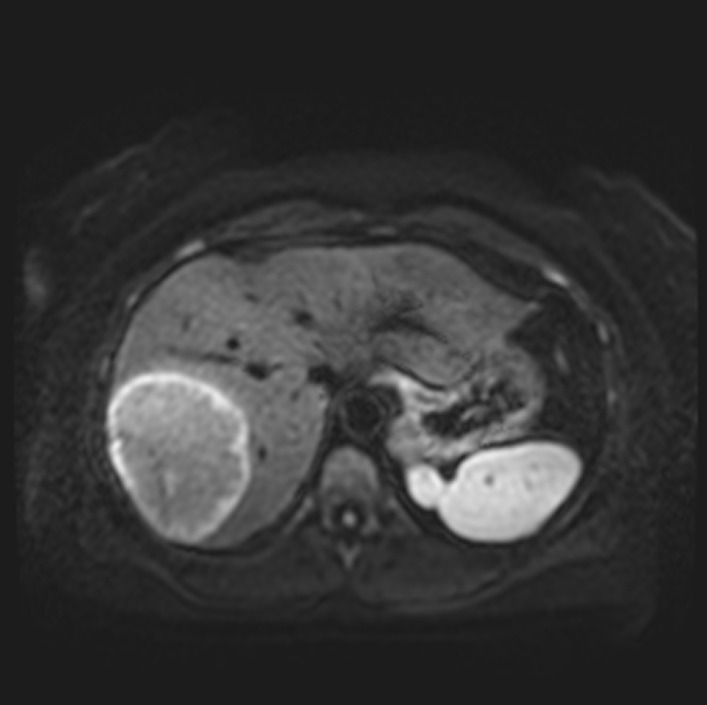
Subsequent abdominal MRI at 6-month follow-up. There is a 9.3 × 7.0 × 9.4 cm circumscribed mass in the right lobe of the liver. It increased substantially in size since 11/07/2019, when it measured 2.3 cm. The mass does not contain lipid. There is a small central cystic or necrotic area. No biliary ductal dilatation and no overlying capsular retraction
